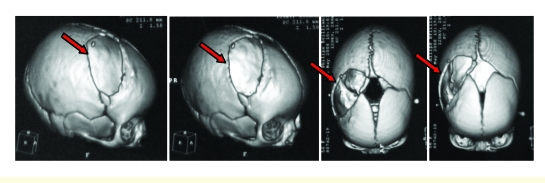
Postoperative 3D reconstruction CT–scan. Frontoparietal craniectomy.
